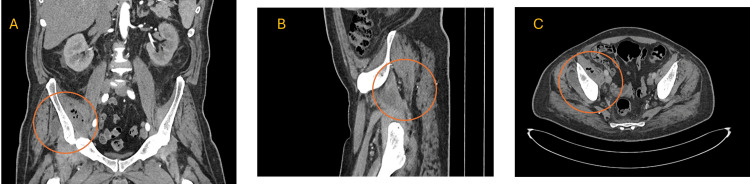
Computed tomography (CT) with IV contrast of the abdomen and pelvis An abdominal and pelvic CT with IV contrast showed large fluid collections with gas locules in the iliac and gluteus minimus muscles. Panel A: Coronal CT image of the abdomen and pelvis. The view shows hypodensity, representing fluid collection with internal gas locules deep to the right iliac and gluteus minimus muscles. Panel B: Abnormal findings in the sagittal CT image. Panel C: Axial CT image of the pelvis. This cross-sectional view provides a better look. The abnormalities include 29 x 44 x 88 mm fluid collections deep to the right iliac muscle and 22 x 38 mm to the right gluteus minimus muscle.
